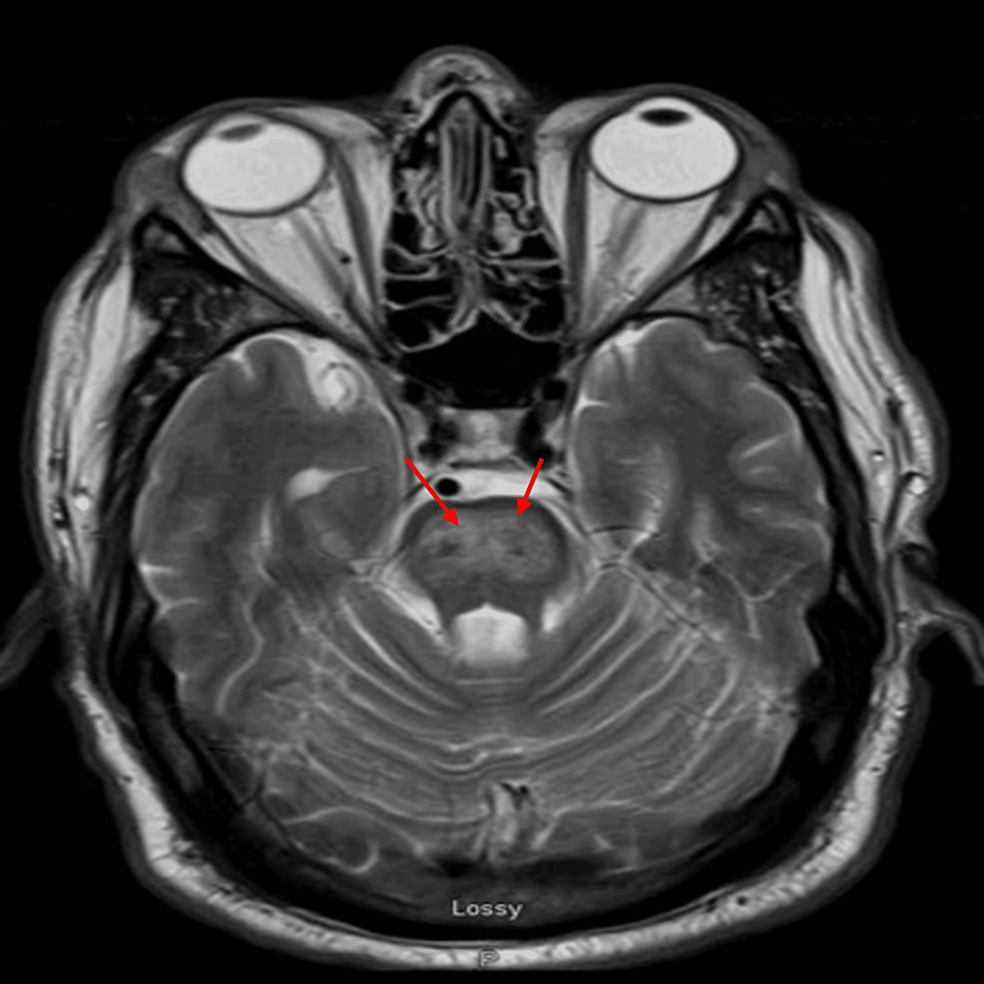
T2-weighted image showing worsening pontine hyperintensities on repeat imaging
